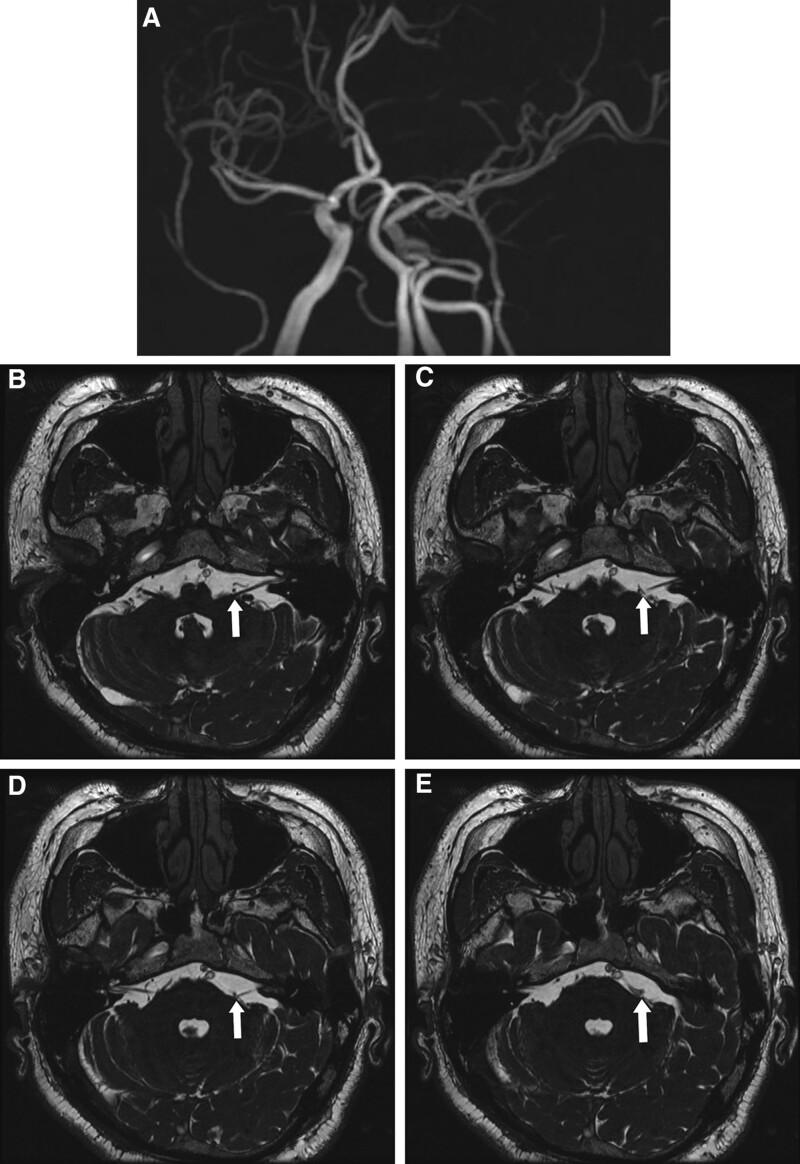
Pre-operative magnetic resonance imaging. (A) Maximum intensity projection magnetic resonance angiography image before surgery. (B–E) Magnetic resonance angiography images showing the neurovascular contact site (white arrows).
